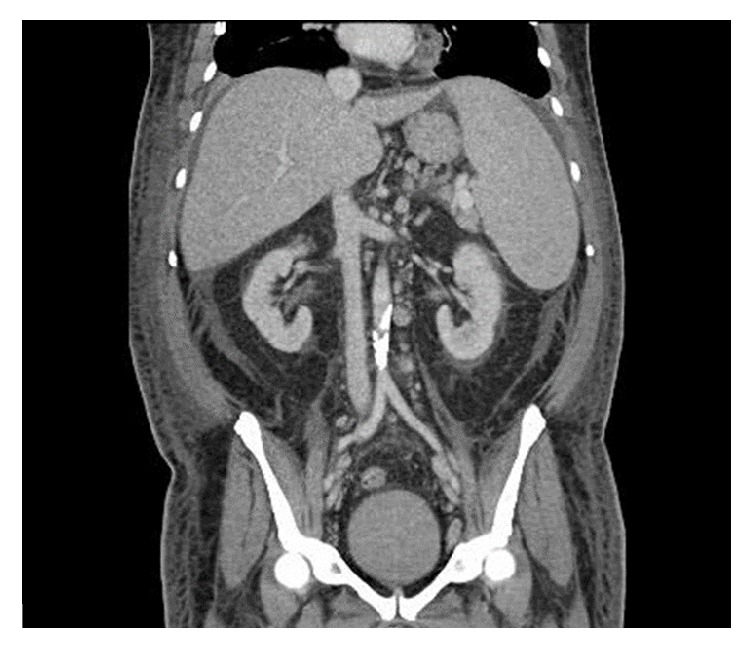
Pretreatment CT with contrast showing enlarged intra-abdominal nodes and splenomegaly.
